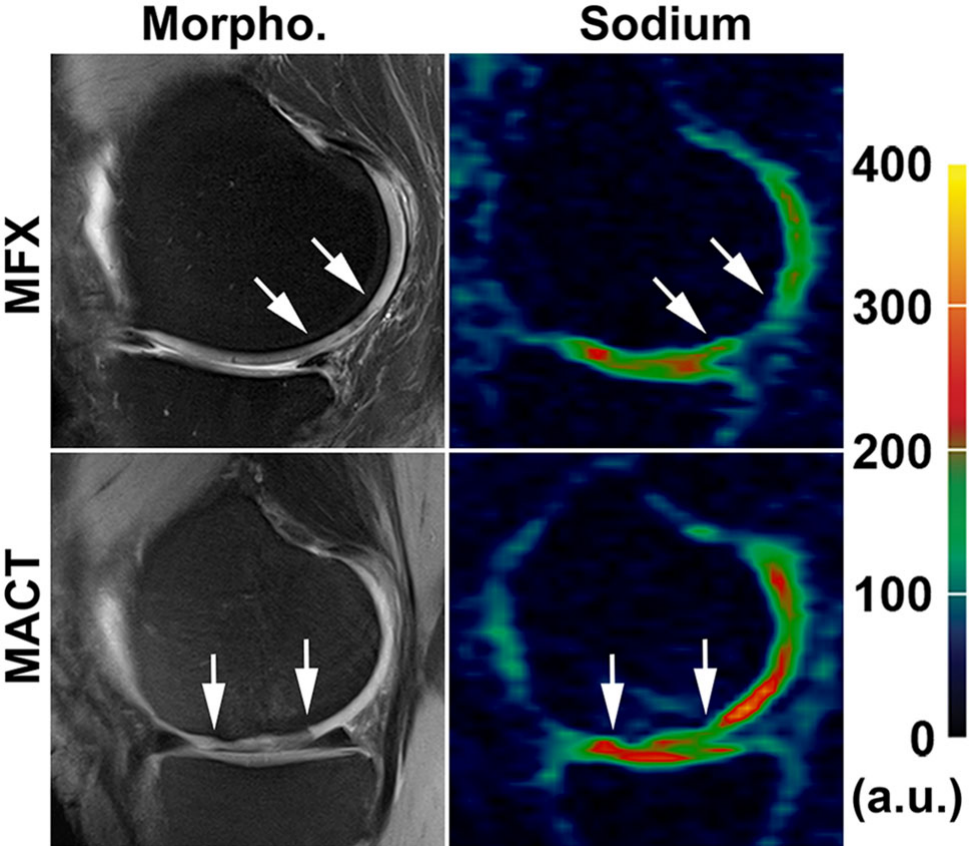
Proton density-weighted MR images with fat suppression (*left column*) and color-coded sodium 3D-GRE images (*right column*) from a patient after MFX treatment (*upper row*) and a subject after MACT surgery (*lower row*). The area of cartilage repair tissue is located between the *arrows*. *Color scale* represents the sodium signal intensity values
